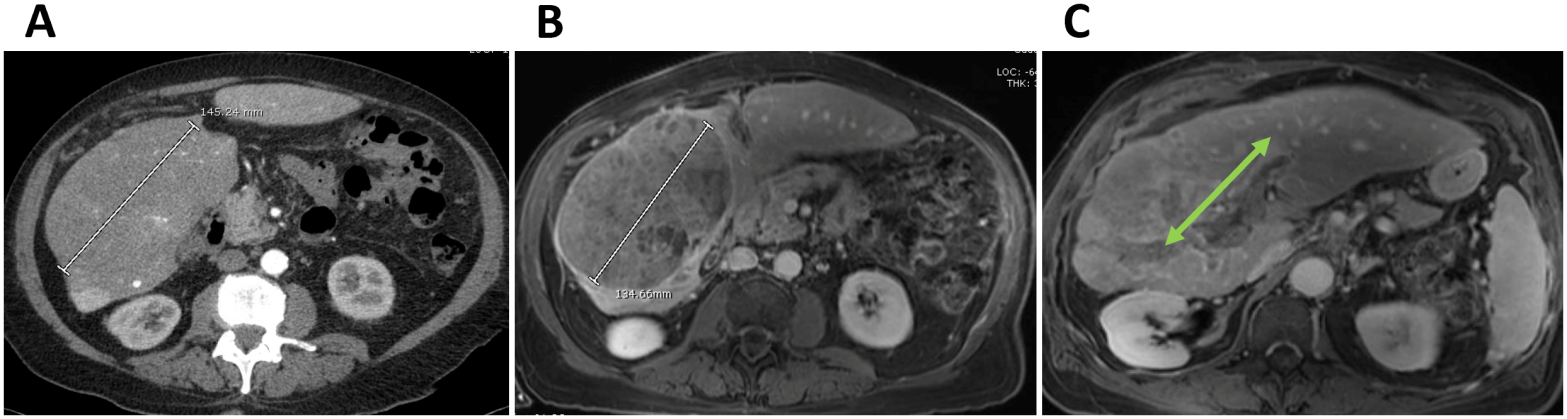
(A) Axial contrast-enhanced CT scan of liver showing the extent of the large HCC throughout the right lobe at diagnosis (AFP 2,838 ng/dL). (B, C) Axial contrast-enhanced T1-weighted MR images after initiation of systemic therapy with atezolizumab-bevacizumab showing stable disease (B) and tumor extension into portal vein branches (arrow) (C).
